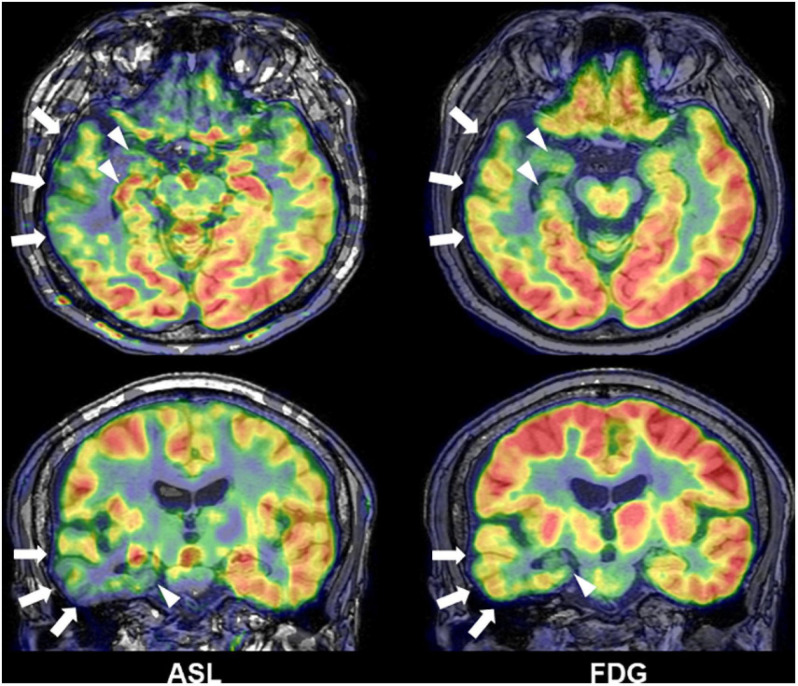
A case of temporal lobe epilepsy with hippocampal sclerosis. Both Arterial spin labeling (ASL) and 18F-FDG PET showed reduced signals around the temporal lobe of focus side (modified from Sone et al., 2019).
